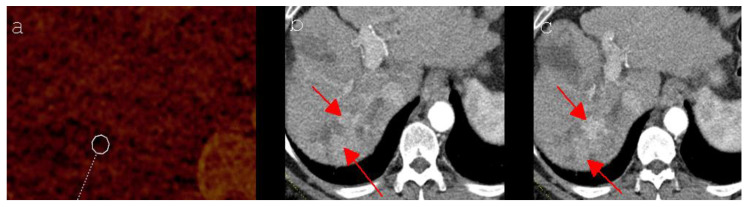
(a) Axial DECT imaging (iodine mapping) shows an ablation zone in segment VII of a 66-year-old man 24 h after MWA of a HCC, including the chosen regions of interest in its peripheral part. (b,c) Axial CT imaging (arterial phase) shows an arterial hypercasvular tumor mass (red arrows) in the peripheral part of the ablated area, which newly occurred at the 12 months follow-up control and turned out to be LTP of a HCC.
